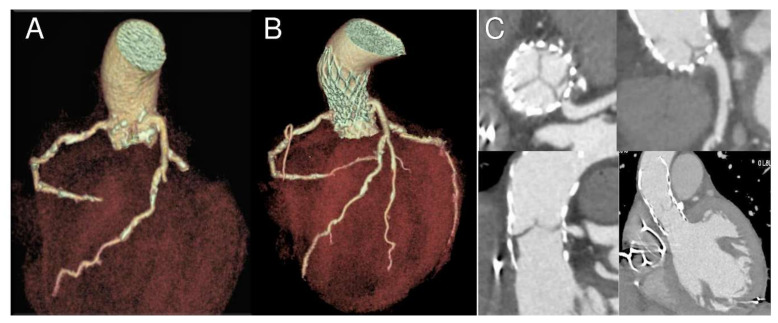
Nine-month follow-up gated CT scan demonstrating bioprosthesis malposition in respect to left main coronary. 3D reconstructions in longitudinal and cross-section before (A) and after the procedure (B,C). Please note coronary ostia proximity with calcifications and ostial stenosis due to partial obstruction by a portion of the THV frame (C).
